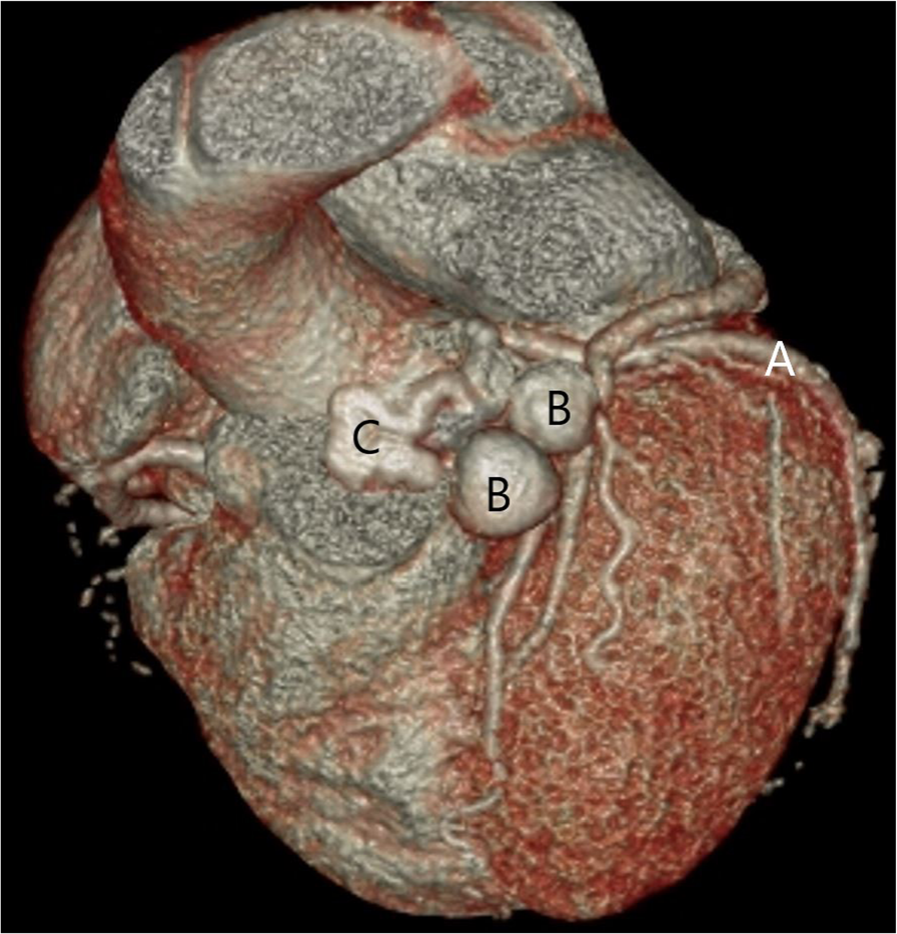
3D reconstruction from a coronary CTA demonstrating: a LADD, b flow related aneurysms, c tortuous artery fistula
